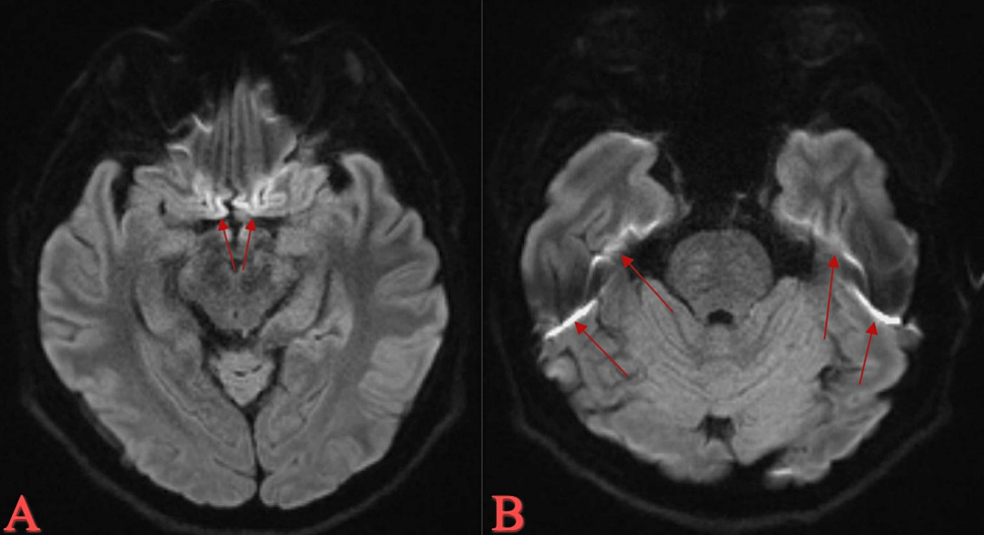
MRI T1 Leptomeningeal enhancement with basilar predominance (A) and of temporal lobes (B).
